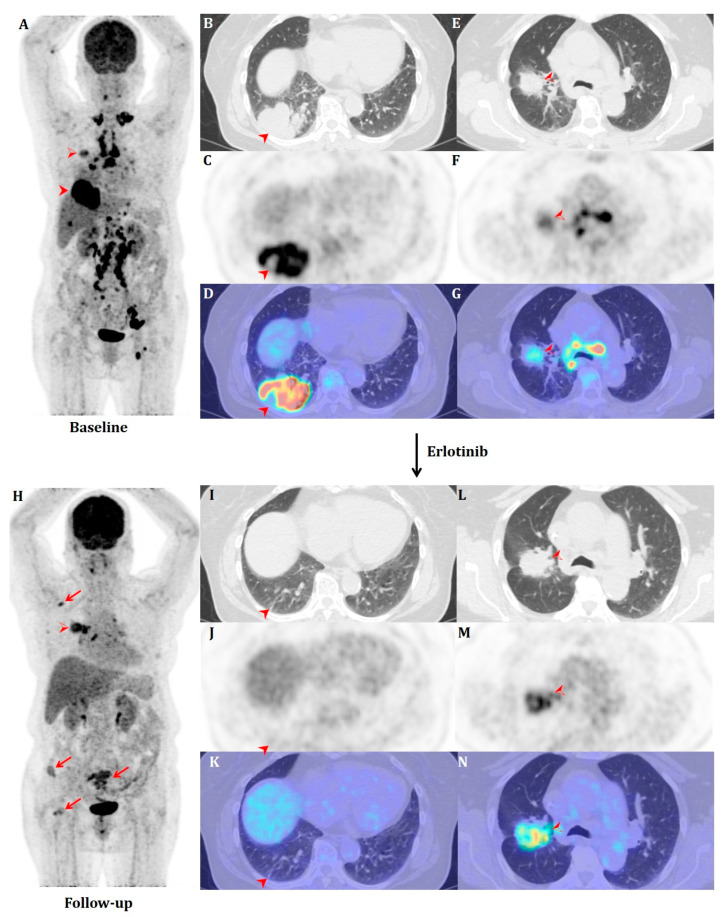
(A) 74-year-old nonsmoker female presenting with chronic cough was referred to our hospital for further evaluation and staging workup of two lung masses which were found on outside CT imaging: a 7.4 cm mass in the RLL abutting the pleura (indicated by arrowheads on (B), CT; (C), PET; and (D), fusion) and a 3.3 cm mass in the RUL with invasion of the right minor fissure (indicated by open arrowheads on (E), CT; (F), PET; and (G), fusion). Extensive metastatic lymphadenopathies were noted on both sides of the diaphragm, as well as liver and bone metastases (T4N3M1c) ((A), MIP). Cytologic diagnosis of bronchial washing of the LLL during bronchoscopy was non-small-cell lung cancer. PCNB of the larger mass proved to be a moderately differentiated adenocarcinoma, while mutation studies came back as EGFR(−)/ALK(+), and the patient was started on alectinib, the highly selective inhibitor of ALK. A subsequent response assessment showed marked regression, followed by stable disease on serial CT follow-up. However, restaging PET/CT a year later (H–N) showed that while the RLL mass was nearly resolved (I–K), the RUL mass (L–N) had in fact progressed, with several newly developed bone metastases in the right scapula, bilateral pelvic bones and right femur (indicated by red arrows on (H), MIP). An additional PCNB showed another moderately differentiated adenocarcinoma, but EGFR (+ for L858R missense mutation)/ALK(−), and the patient was switched to erlotinib, a classical EGFR tyrosine kinase inhibitor. Follow-up CT three months later showed marked regression of the RUL mass, but redevelopment of multiple abdominal nodes. The patient was then switched to lorlatinib to again cover the ALK rearrangement. Extrathoracic metastases showed complete response, but both primary tumors progressed two months later.
